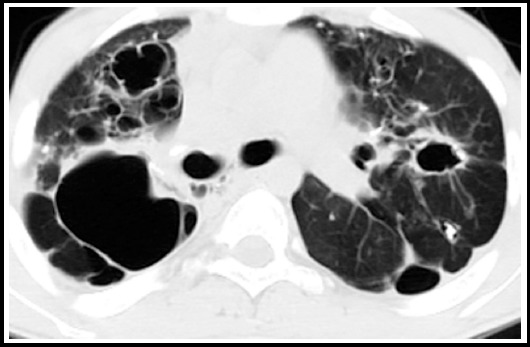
CT chest with IV contrast. Lung and mediastinal windows show tuberculoma in left upper lobe.
